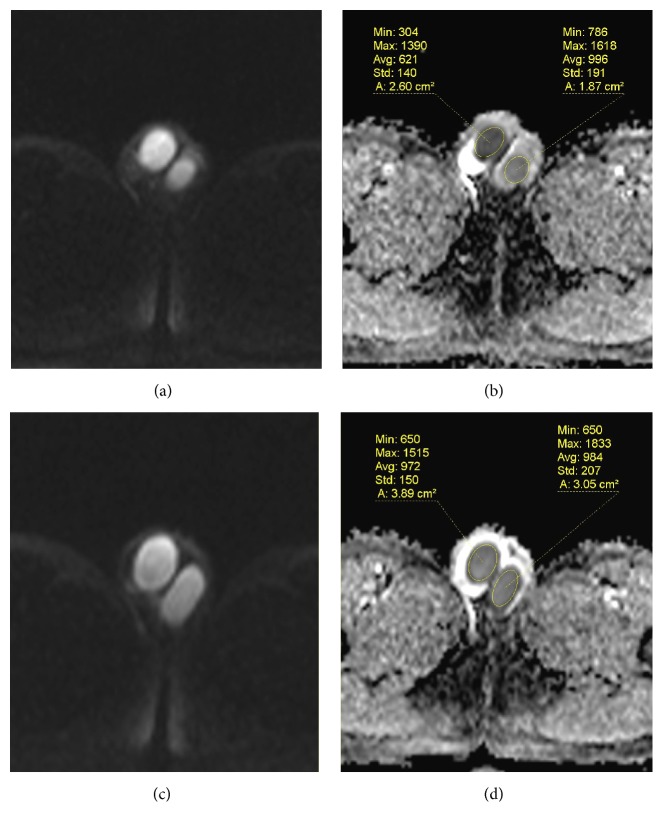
The right testicle shows high signal intensities on DWI (a). In the ADC map (b), the right testicle demonstrates lower ADC value than the left testicle. On DWI (c) and ADC map (d) obtained half an hour later following the preoperative external detorsion procedure, an isointense right testicle relative to the unaffected left testicle appears, which reflects the restoration of parenchymal blood flow.
